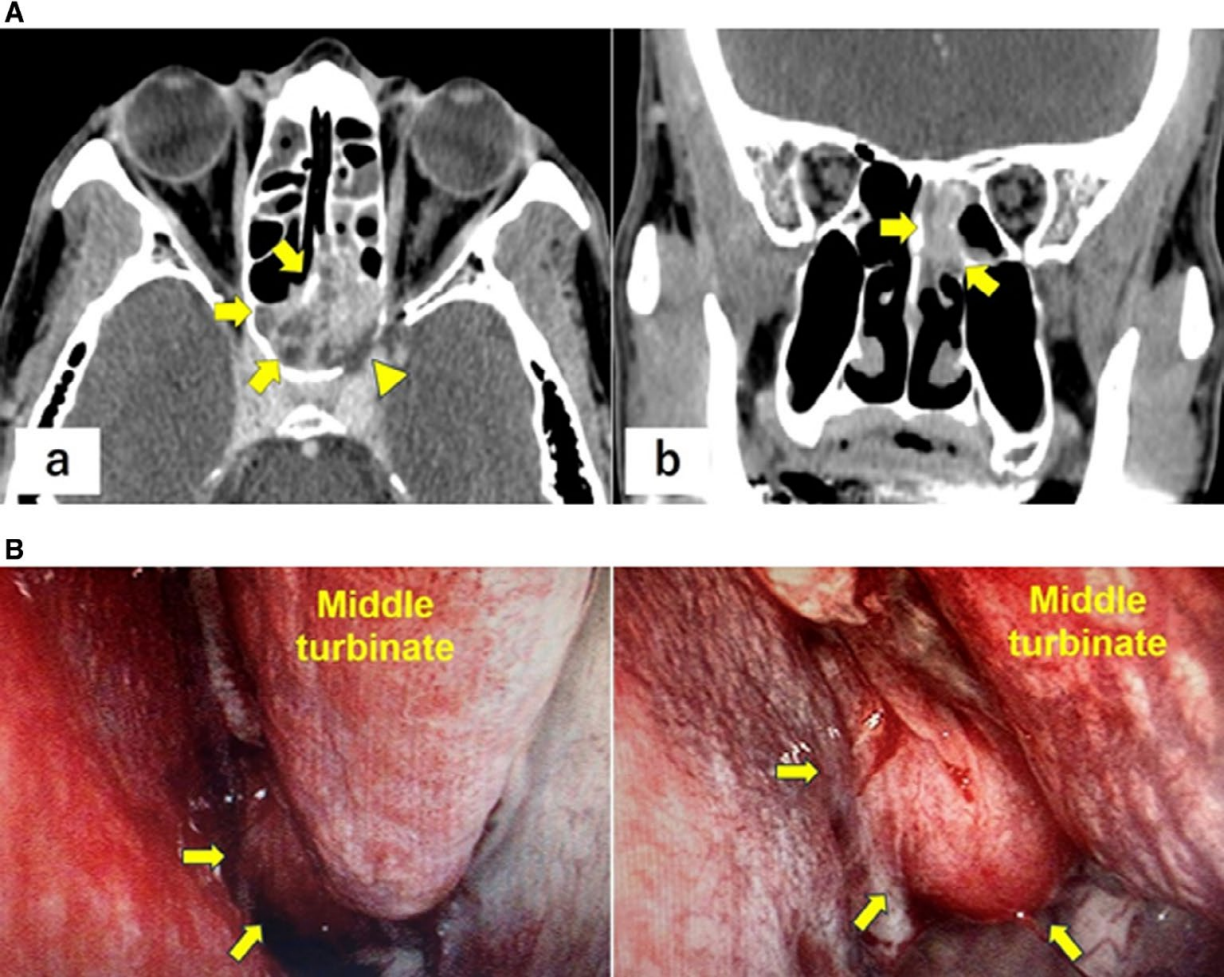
FIGURE 1 (A) Contrast‐enhanced head computed tomography images at presentation demonstrating an approximately 4‐cm hypervascular mass centered in the sphenoid sinus with osseous destruction involving the sellar floor and clivus. (a) Axial image. (b) Coronal image. Arrows: tumors; arrowheads: osseous destruction. (B) Nasal endoscopic view demonstrating a tumor located posterior to the left middle turbinate. Arrows: tumors.
